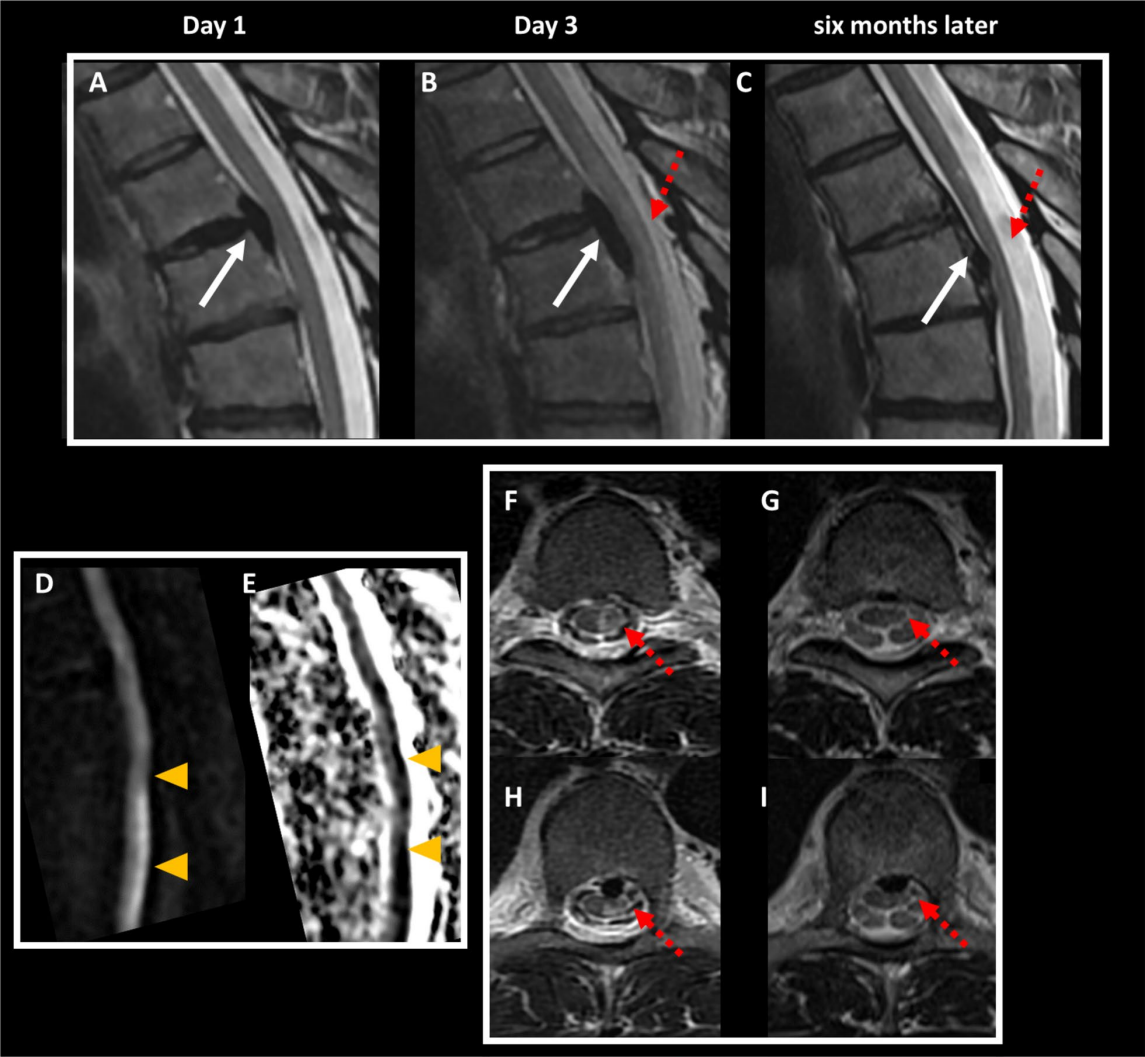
Evolution of MRI findings over time. Initial MRI scan showed a disc extrusion at the T5–T6 level (arrows) without any spinal cord signal abnormality (A). The MRI performed 2 days later showed an increase in size of the disk extrusion and the appearance of an extensive spinal cord edema (dashed arrows) (B) involving the left lateral half of the cord as seen on axial T2-weighted images (dashed arrows) (F, G). Diffusion-weighted images revealed a moderate area of hyperintensity (D) with a low apparent diffusion coefficient (arrowheads) (E) in favor of an ischemic process. Follow-up MRI 6 months later showed a partial regression of the disc herniation (arrows) and a decrease of the signal abnormalities with the appearance of an atrophy (dashed arrows) (C, H, I)
